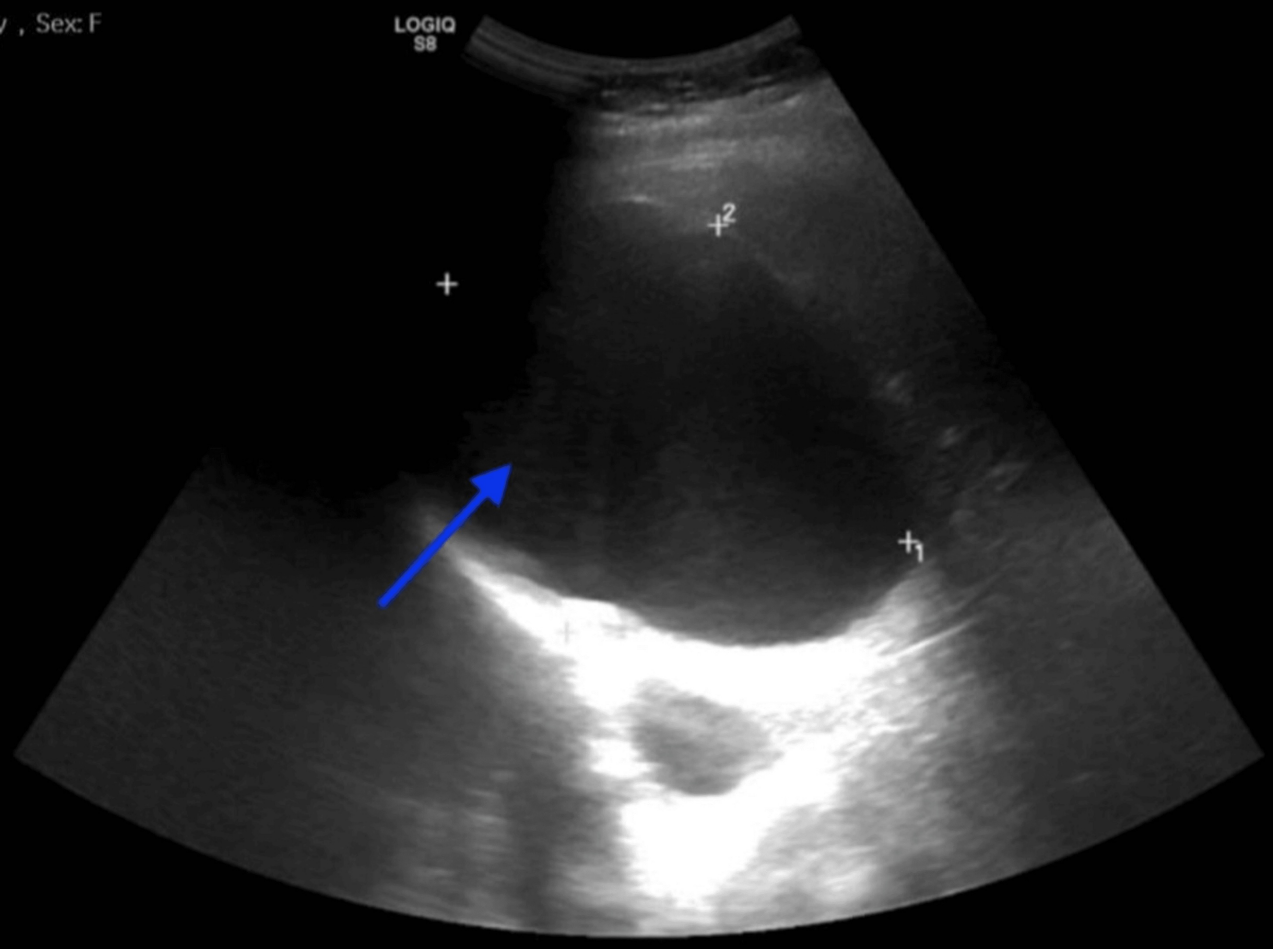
Abdominal Ultrasound Showing Hepatomegaly of the Right lobe of the Liver Especially Segment VIII with a Sizable Simple Cystic Lesion Measuring 9.7×7.9×7.3 cm (Blue Arrow).
